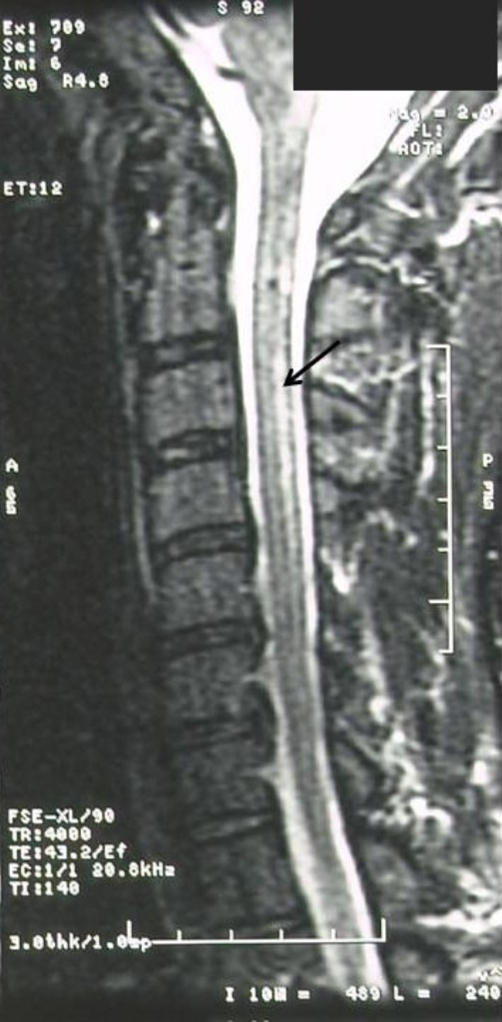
Flamed-shaped area of increased signal intensity within the posterior column of the cervical spine on the sagittal T2 weighted MRI.
